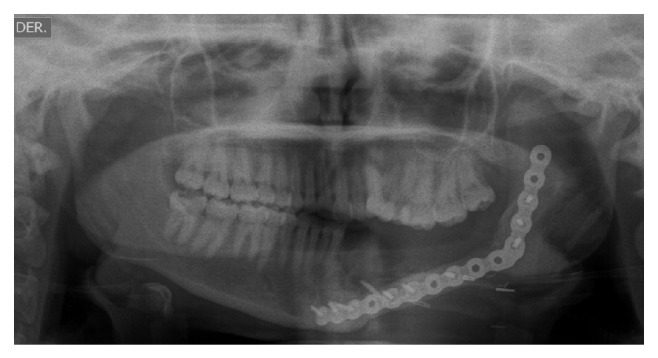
Panoramic radiograph showing area of mandibular resection with no evidence of recurrence 1 year after surgery.
